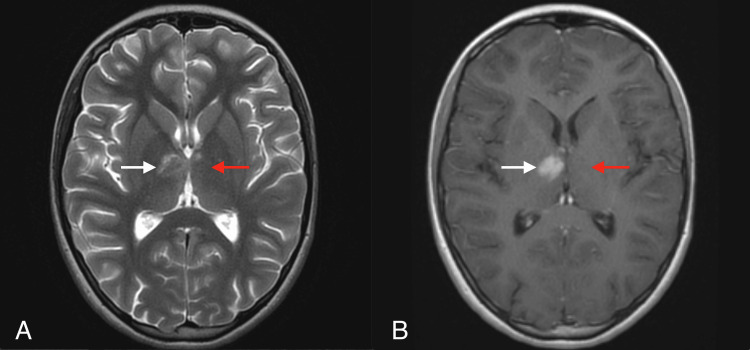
Day 12 from symptom onset. Brain MRI performed in the Pediatric Neurology Department demonstrates evolution of stroke lesions: right thalamic infarct (white arrow) and small punctate changes in the left thalamus (red arrow) on T2-weighted (A) and post-contrast T1-weighted with trace enhancement (B) images.
